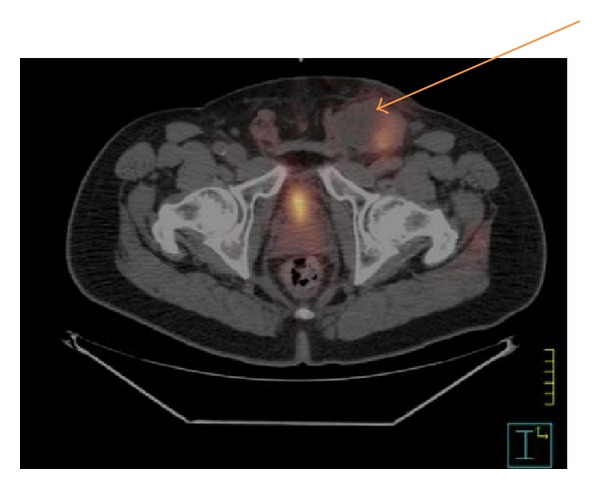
Initial staging PET-CT revealed SUV uptake at the base of the penis (not shown) as well as SUV uptake in a left inguinal lymph node (shown above).
